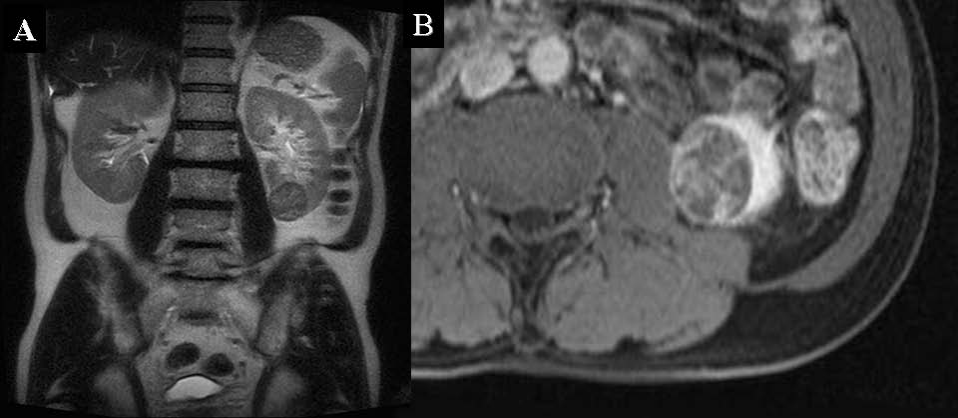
CT images. (A): A 3 cm poorly enhanced, well-defined mass was observed in the inferior pole of the left kidney on CT. (B): A 2 × 2 × 3 cm well-defined, solid tumor with a white capsule was detected in the inferior pole of the left kidney at operation. Inside the mass, hematoma and necrotic tissue were partly observed. Neither obvious enlargement of lymph nodes nor metastasis to intraperitoneal organs was seen.
